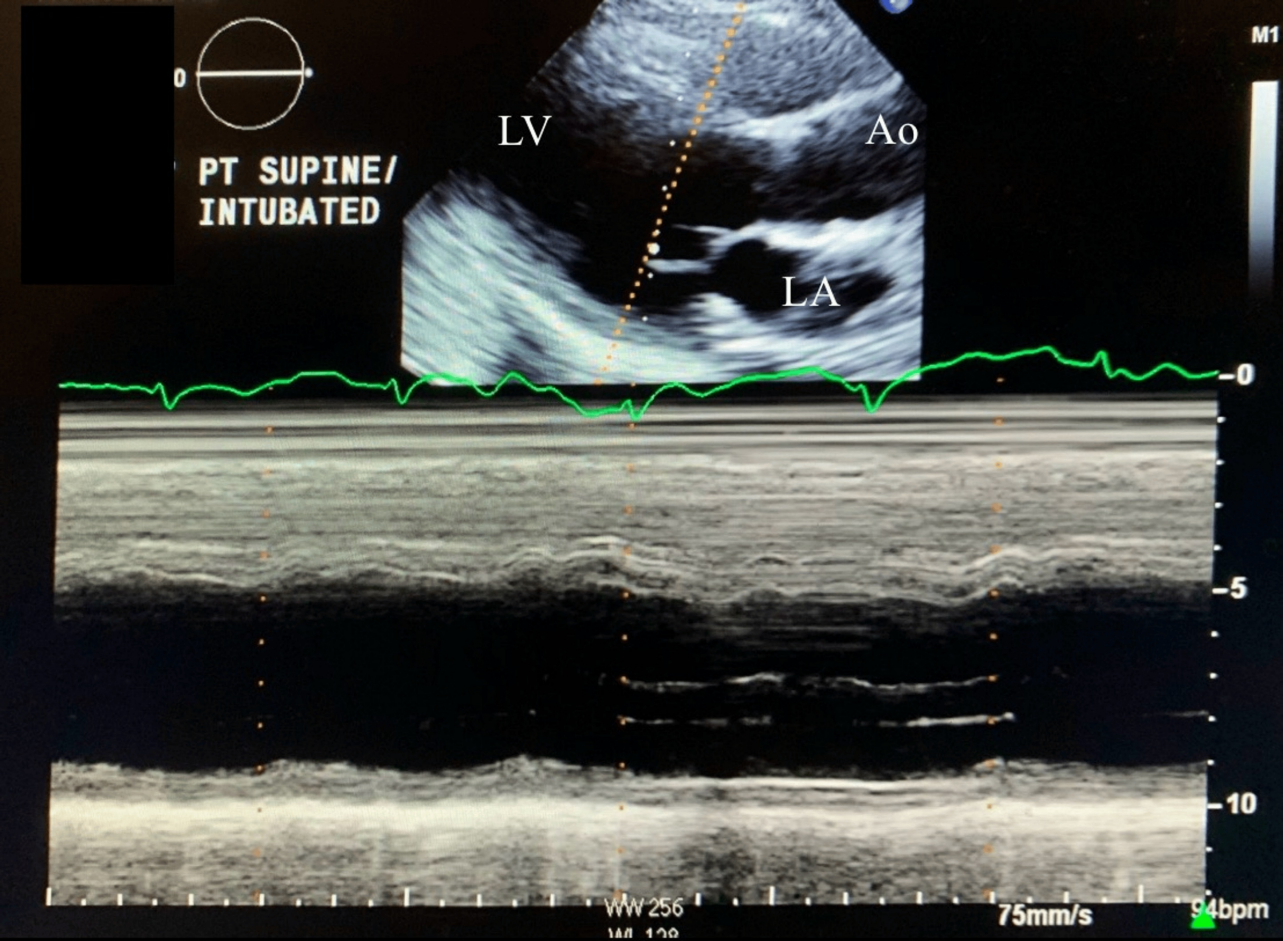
M mode echocardiography from parasternal long axis view showing ejection fraction of 10-15% on day one of admission. LV: left ventricle; LA: left atrium; Ao: aorta
